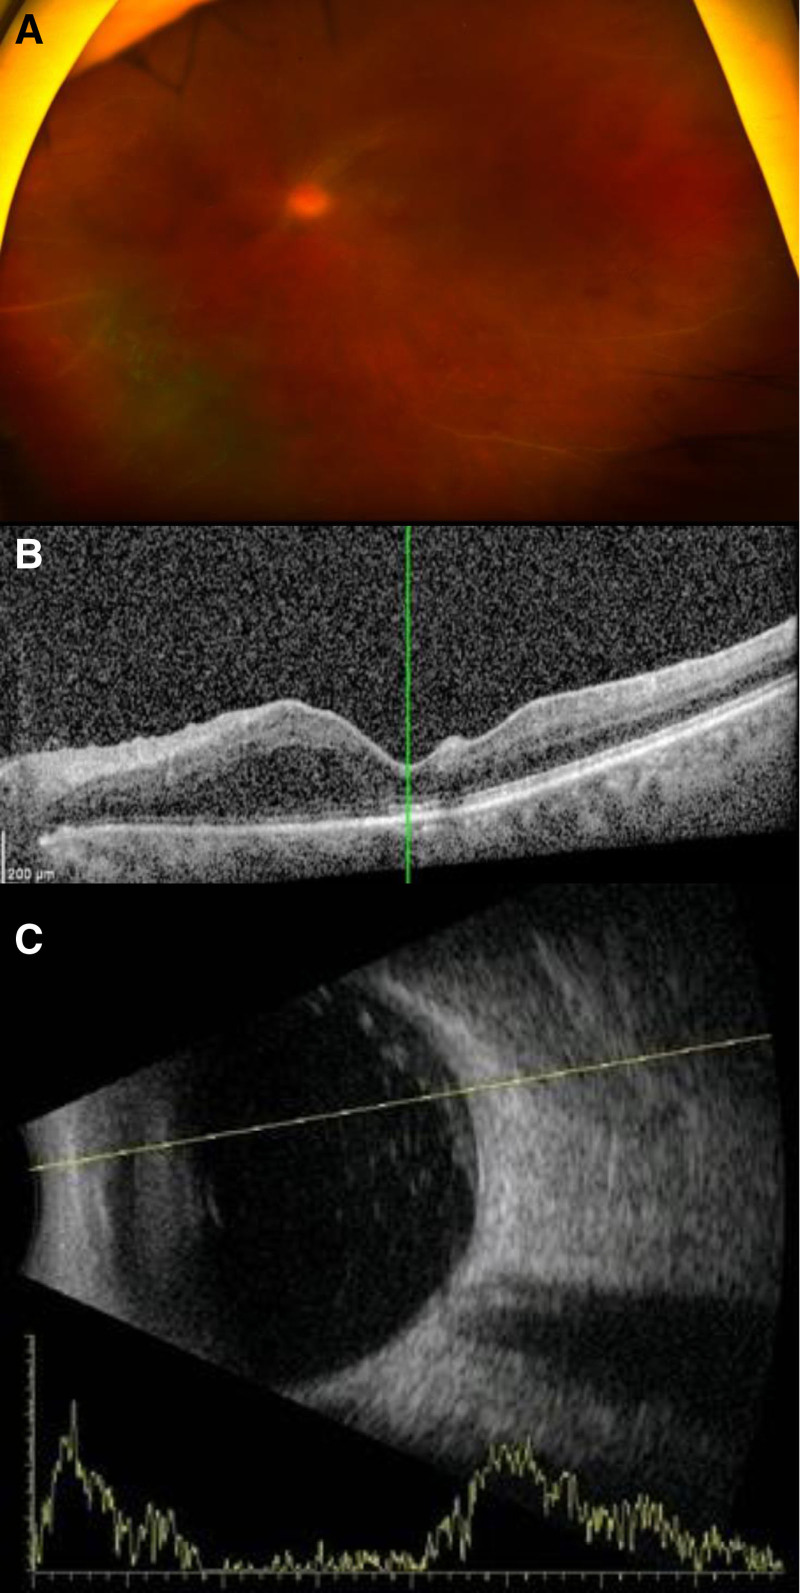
Two days after intravitreal ceftazidime, vancomycin, and ganciclovir injections in the right eye, the patient developed blurred vision in the left eye. (A) Fundus examination reveals vitritis and multiple retinal hemorrhages and exudation in the left eye. (B) Outer retinal edema of the macula of the left eye was observed on optical coherence tomography. (C) B-scan ultrasonography confirms vitreous opacification in the left eye.
